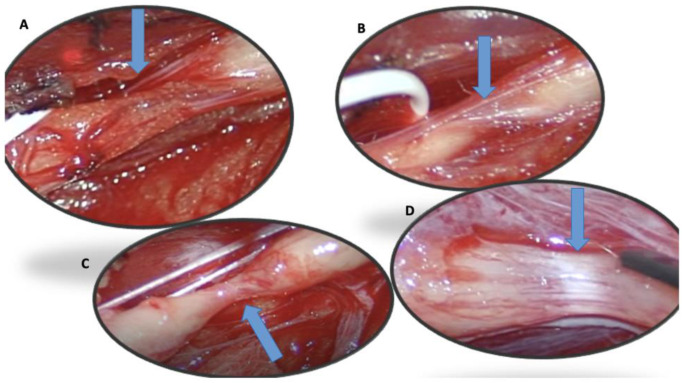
(A–D): Four torsion sites described sonographically in case 1 and confirmed surgically (blue arrow).
